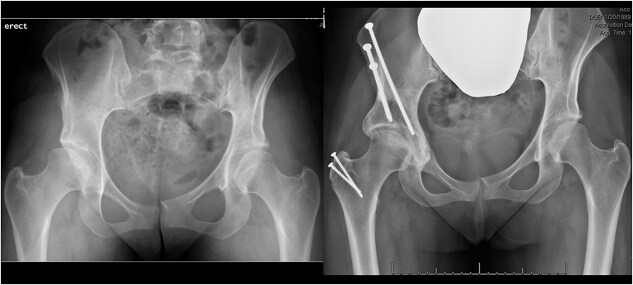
Representative pre-op and post-op radiographs of a patient who underwent combined OATS and PAO. Left: Pre-op AP pelvis. Right: One year post-op AP pelvis.
